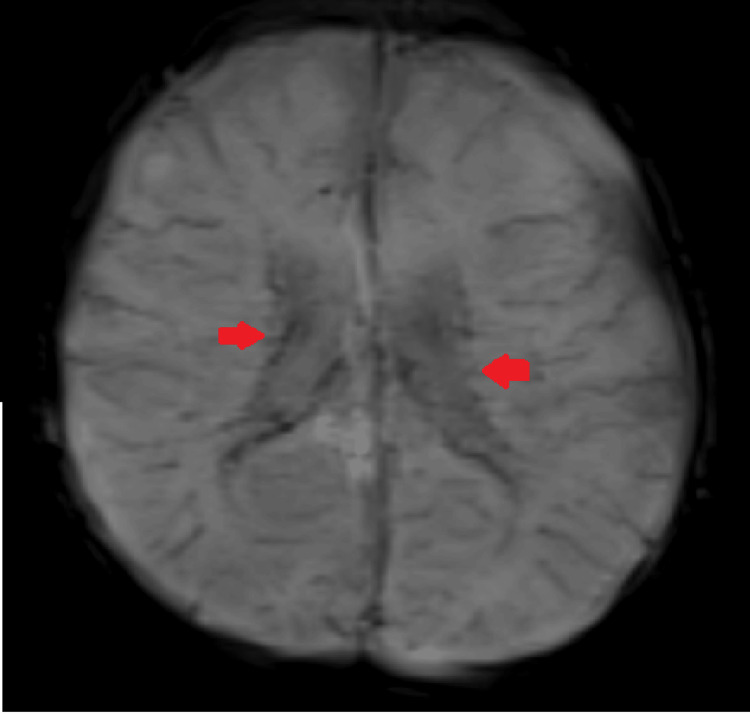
Susceptibility-weighted image (SWI) showed multiple, tiny blooming areas in periventricular regions, which concurred with the calcifications previously seen on CT
